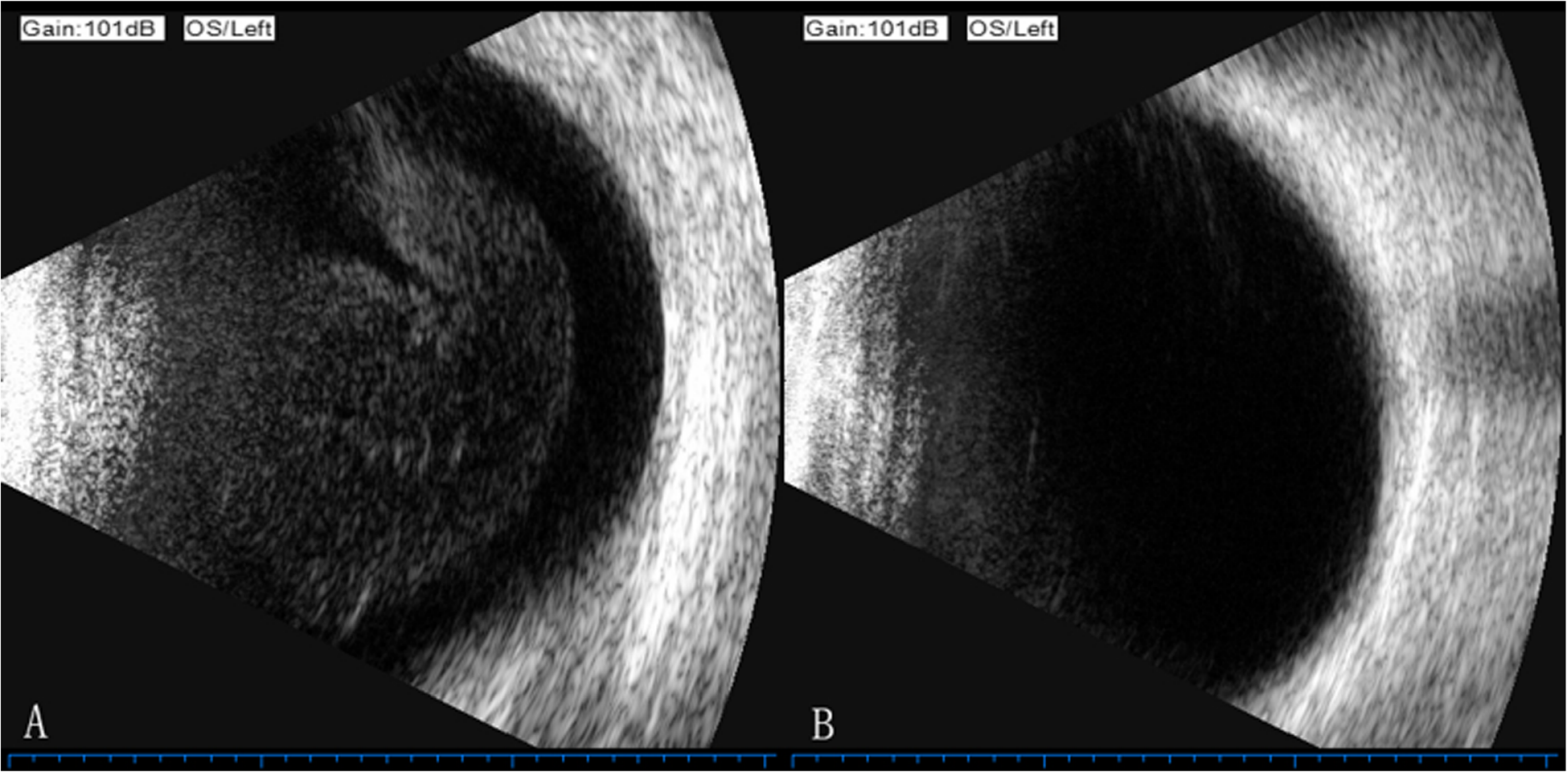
B-ultrasound scan exam of the left eye of patient 18. a Exam performed before the initiation of treatment with methotrexate. Note the numerous, homogeneous collections of medium-amplitude mobile echoes in the anterior and middle segments of the vitreous. b Exam performed after the 8th (1 month) methotrexate injection. Note the clearing o the vitreous cavity
